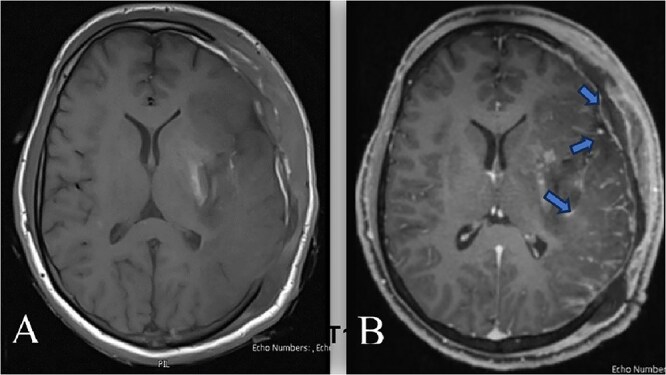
Post- craniectomy T1 weighted MRI head axial view. (A) Pre- gadolinium contrast (B) post- gadolinium contrast: Postcontrast left hemispheric prominent superficial vascular and leptomeningeal enhancement.
